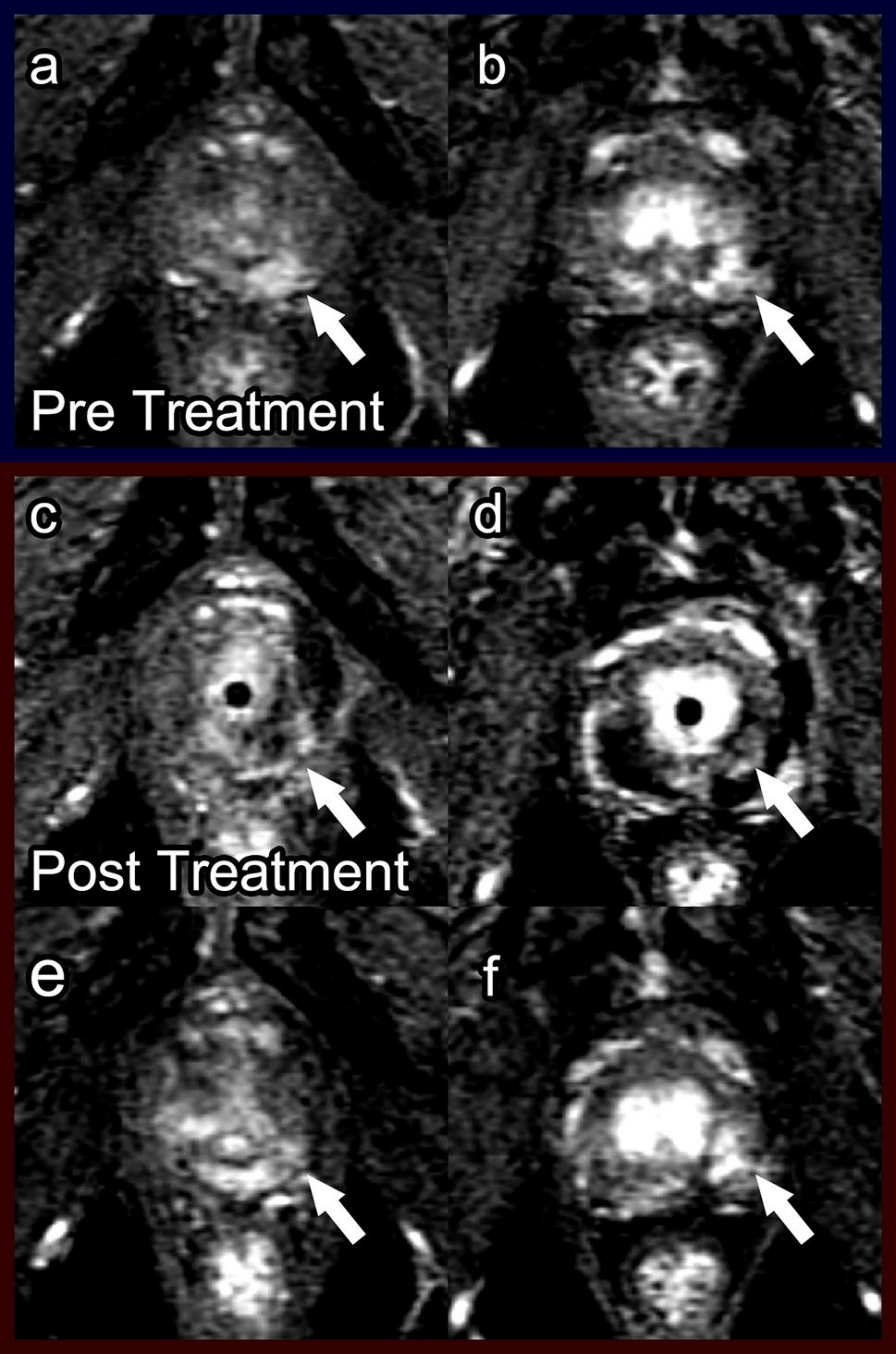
Low-grade recurrence after photodynamic therapy for recurrent prostate cancer after external beam radiation therapy. Pre-treatment prostate MRI: Fat-suppressed DCE during wash-in in the first 30s post-contrast injection (a, b) showed focal enhancement indicative of tumor in the left mid and apical posterior medial and lateral peripheral zone (arrow). Biopsy in this region showed Gleason score 4 + 3 prostate cancer in 20% and 30% of cores. Bilateral laser fibers were placed in the prostate and activated after injection of WST09 (TOOKAD) as part of a research protocol [23]. Post photodynamic therapy prostate MRI at 7 days: DCE (c, d) showed bilateral loss of enhancement in the posterior peripheral zone. There were a few tiny spots of residual enhancement (arrow) in the tumor region. Post photodynamic therapy prostate MRI at 6 months: DCE (e, f) showed increased enhancement in the treated areas (arrow). Biopsy showed low-grade cancer only (Gleason score 3 + 3 in 20% and 5% of the cores) in the treated region
